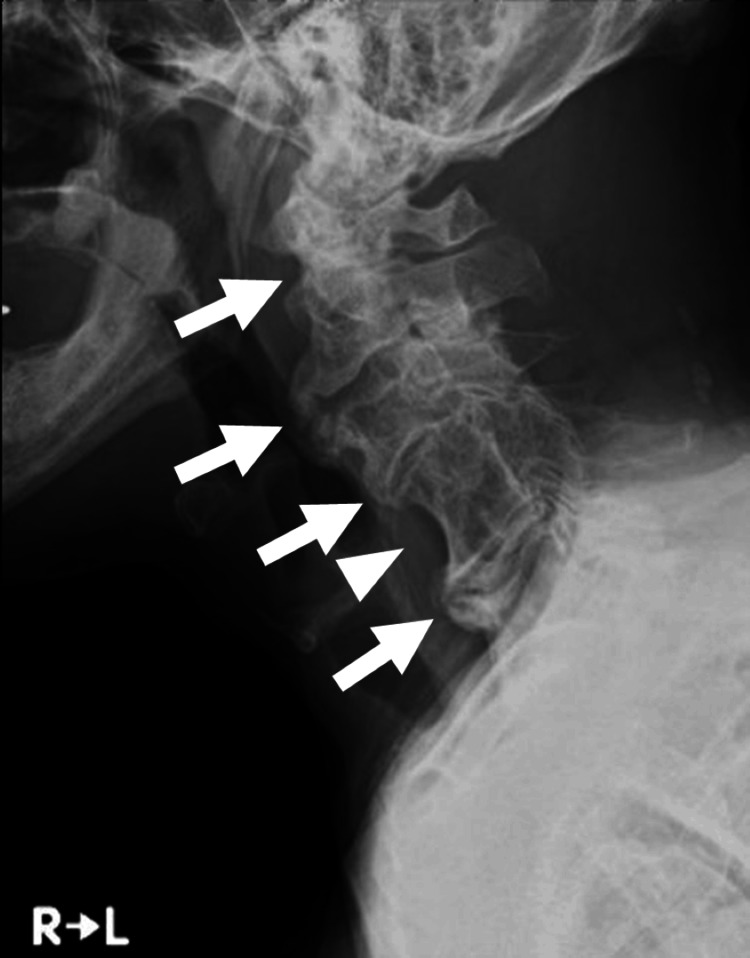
Plain X-ray of the cervical spine Degenerative changes are seen across multiple cervical vertebrae (arrow). Vertebral body fusion is observed at the C4-C5 level (arrowhead).
